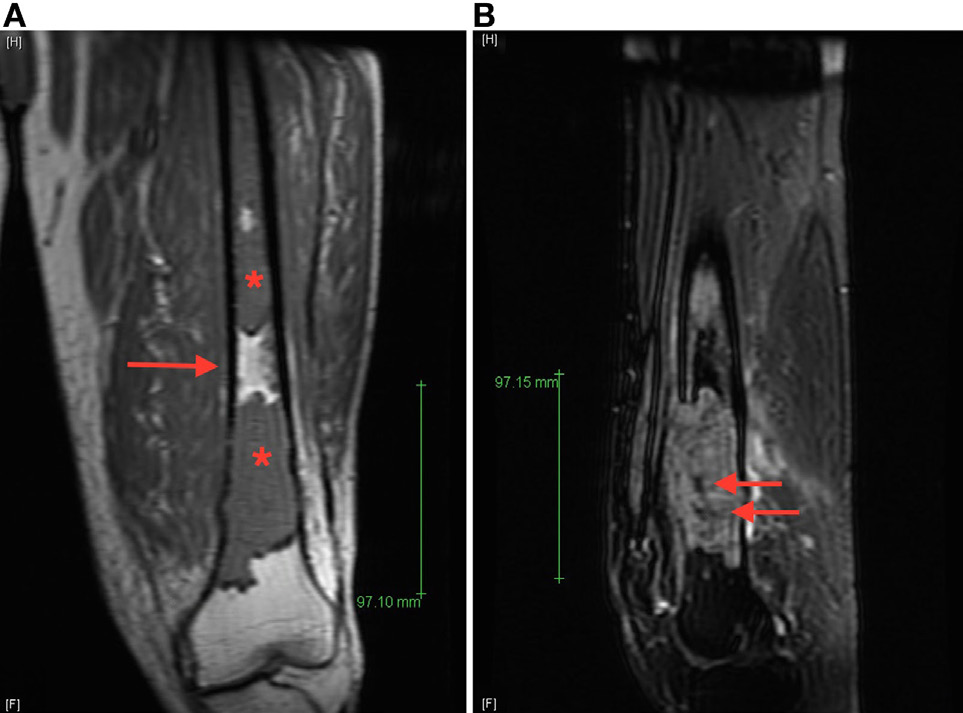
(A) Coronal T_1_ magnetic resonance imaging (MRI) image of the left femur demonstrates T_1_ hypointense lesions (starts) with normal intervening bone marrow (arrow), (B) sagittal T_2_ fat-saturated MRI image of the dominant distal femur lesion demonstrated intermediate T_2_ hyperintensity with areas of marked low signal intensity centrally (arrows), suggestive of areas of sclerosis not appreciated radiographically.
